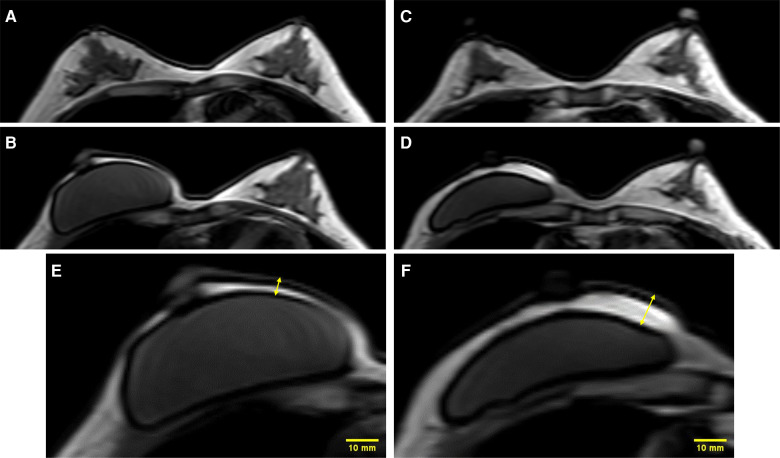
Difference in mastectomy flap thickness between c-NSM and m-NSM on MRI images: (A) preoperative MRI image of the patient with right breast cancer; (B) one-month postoperative MRI image of the same patient after c-NSM and IBR using implant; (C) preoperative MRI image of another patient with right breast cancer; (D) postoperative MRI image after m-NSM and IBR using implant at 1-month follow-up; (E) 2x image of the breast shows the flap thickness of 4.92 mm after c-NSM; (F) flap thickness was 10.23 mm after m-NSM. c-NSM, conventional Nipple Sparing Mastectomy; m-NSM, modified Nipple Sparing Mastectomy.
